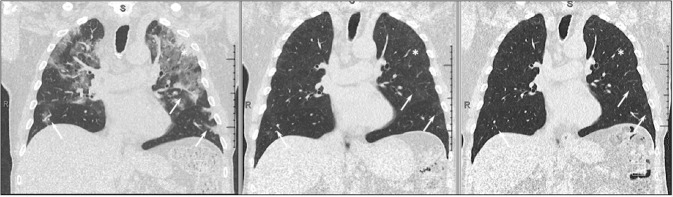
Typical SARS-CoV-2 CT scan lesions and their evolution at 3 and 12 months. Asterisks show complete healing of the areas of consolidation at 3 months. White arrows demonstrate a slower but almost complete resolution of the areas of ground glass opacities at 3 and 12 months.
